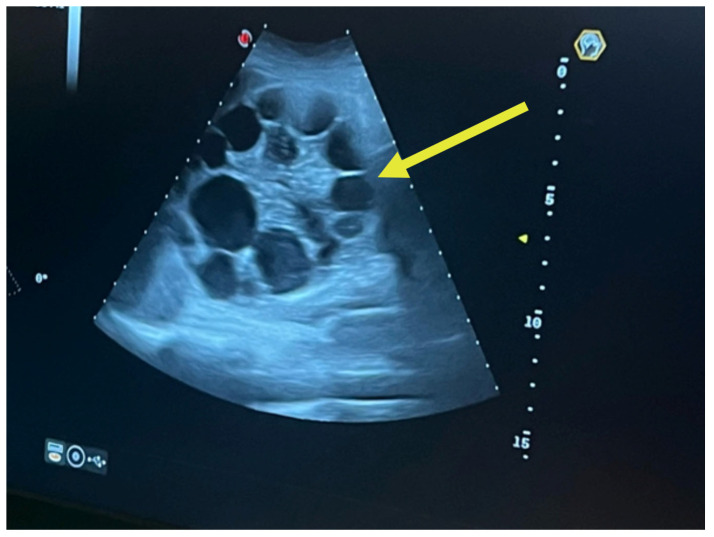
Intraoperative ultrasound revealing suspected renal-origin hydatid cyst (third lesion): the scan reveals a complex, multiloculated cystic structure with multiple anechoic (black) daughter cysts contained within a well-defined parent cyst.
